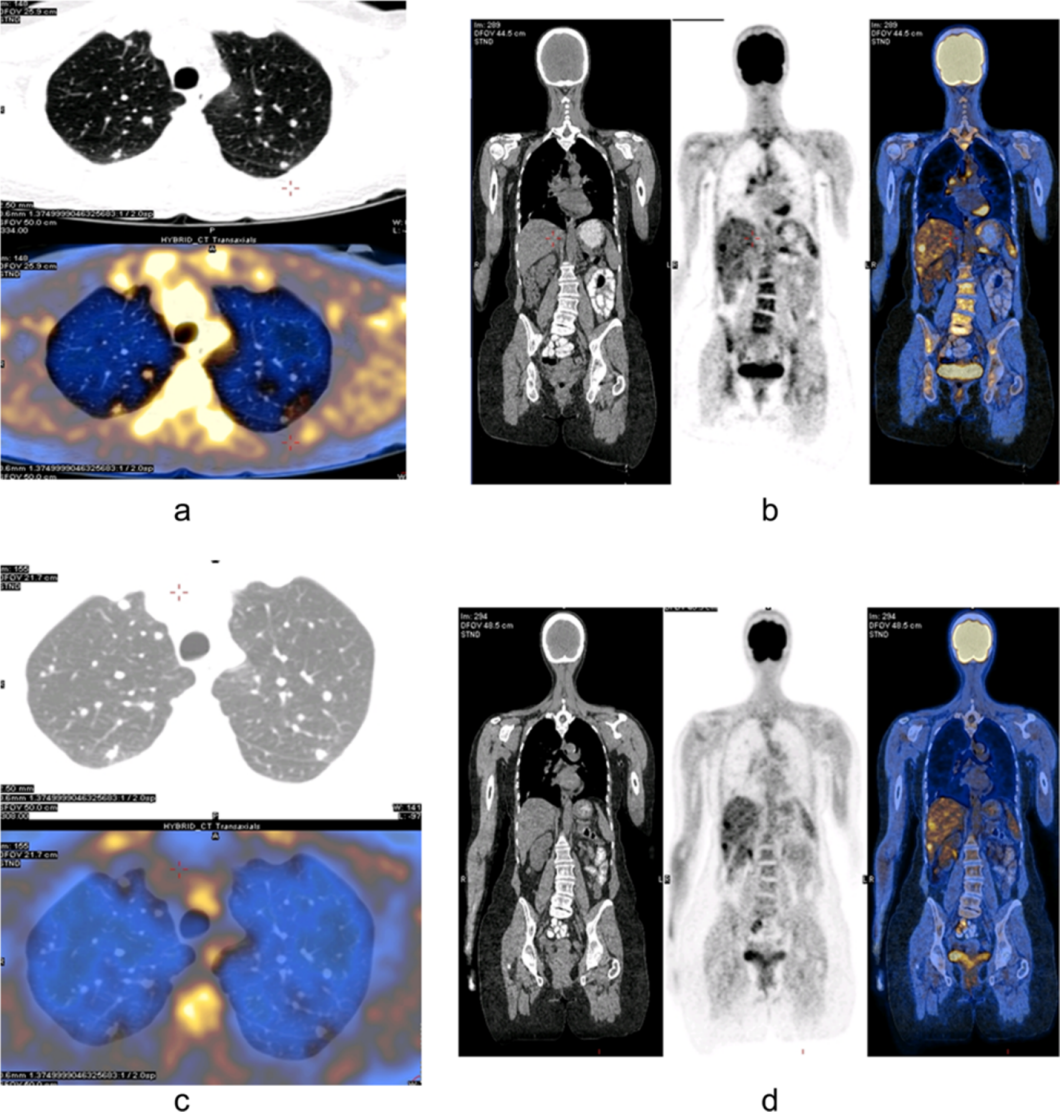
18F-FDG PET-CT. a PET-CT (selected axial slice) performed at staging, demonstrates pathological FDG foci in a few lung nodules. b PET-CT (selected coronal slice) performed at staging, demonstrates pathological FDG foci in mediastinal lymph nodes and the liver. Additional findings were demonstrated in a few cervical lymph nodes (not shown). c PET-CT (selected axial slice) performed after treatment, demonstrates a few lung nodules with no FDG uptake. d PET-CT (selected coronal slice) performed after treatment, demonstrates pathological FDG foci in the liver. No mediastinal findings are shown
